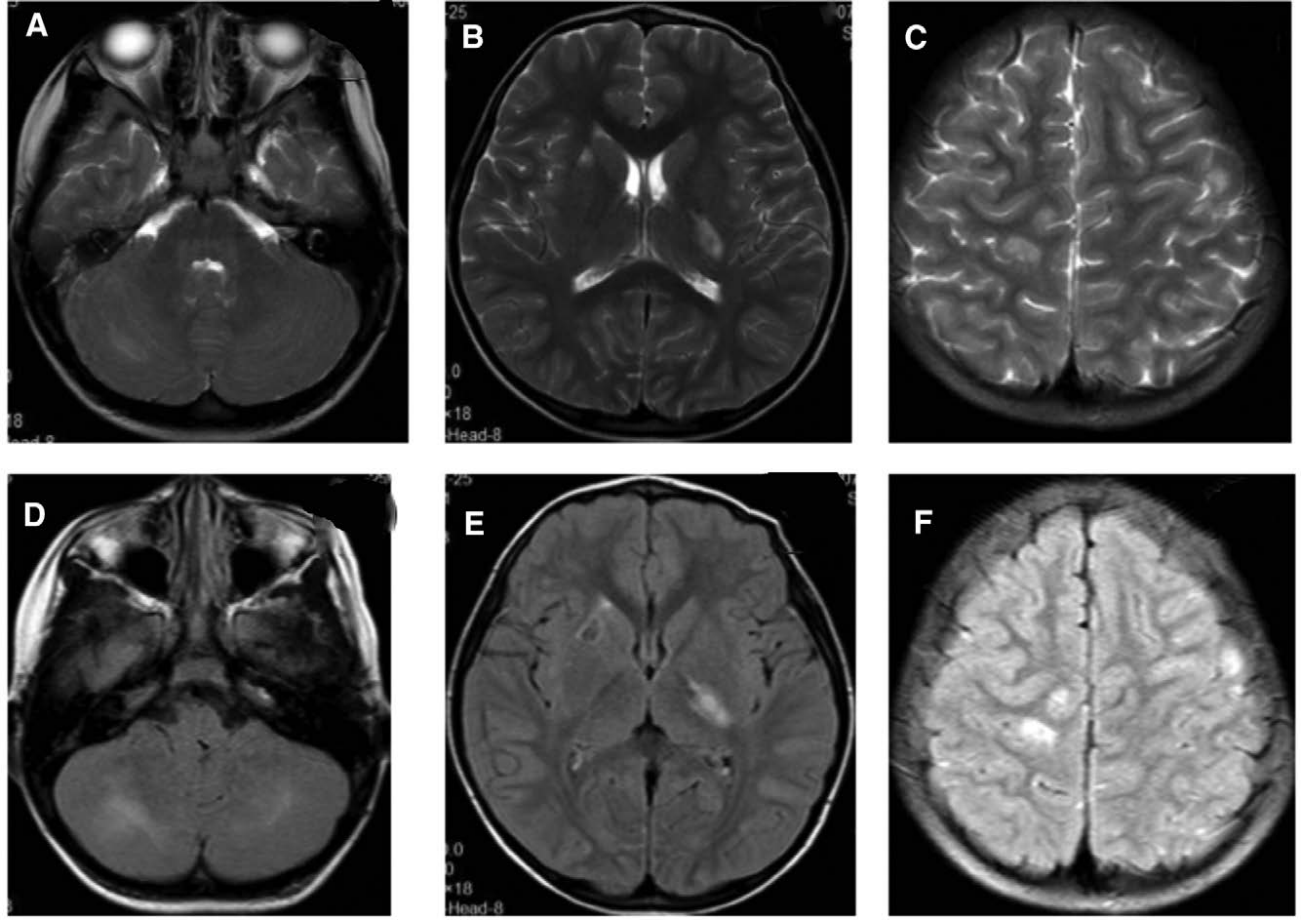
Cranial MRI of Case 2 at the age of 4 years and 11 months. Multiple abnormal signals found in bilateral cerebellar hemispheres, left basal ganglia, left temporal, and bilateral parietal regions. In addition, the encephalomalacia foci at the right basal ganglia could not be ruled out. MRI = magnetic resonance imaging.
